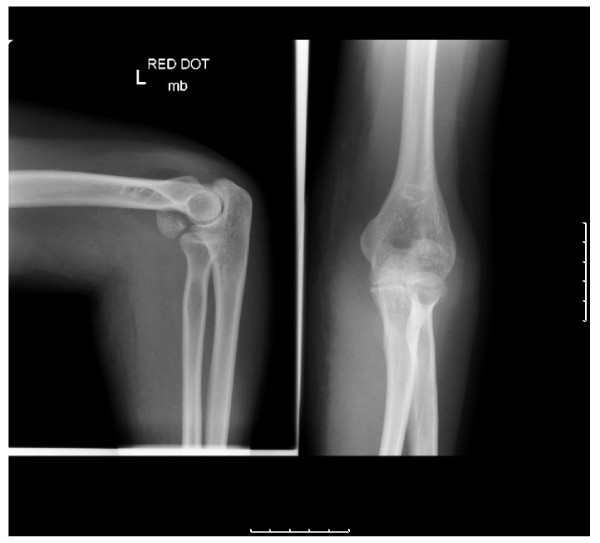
Radiograph of capitellar fracture.
